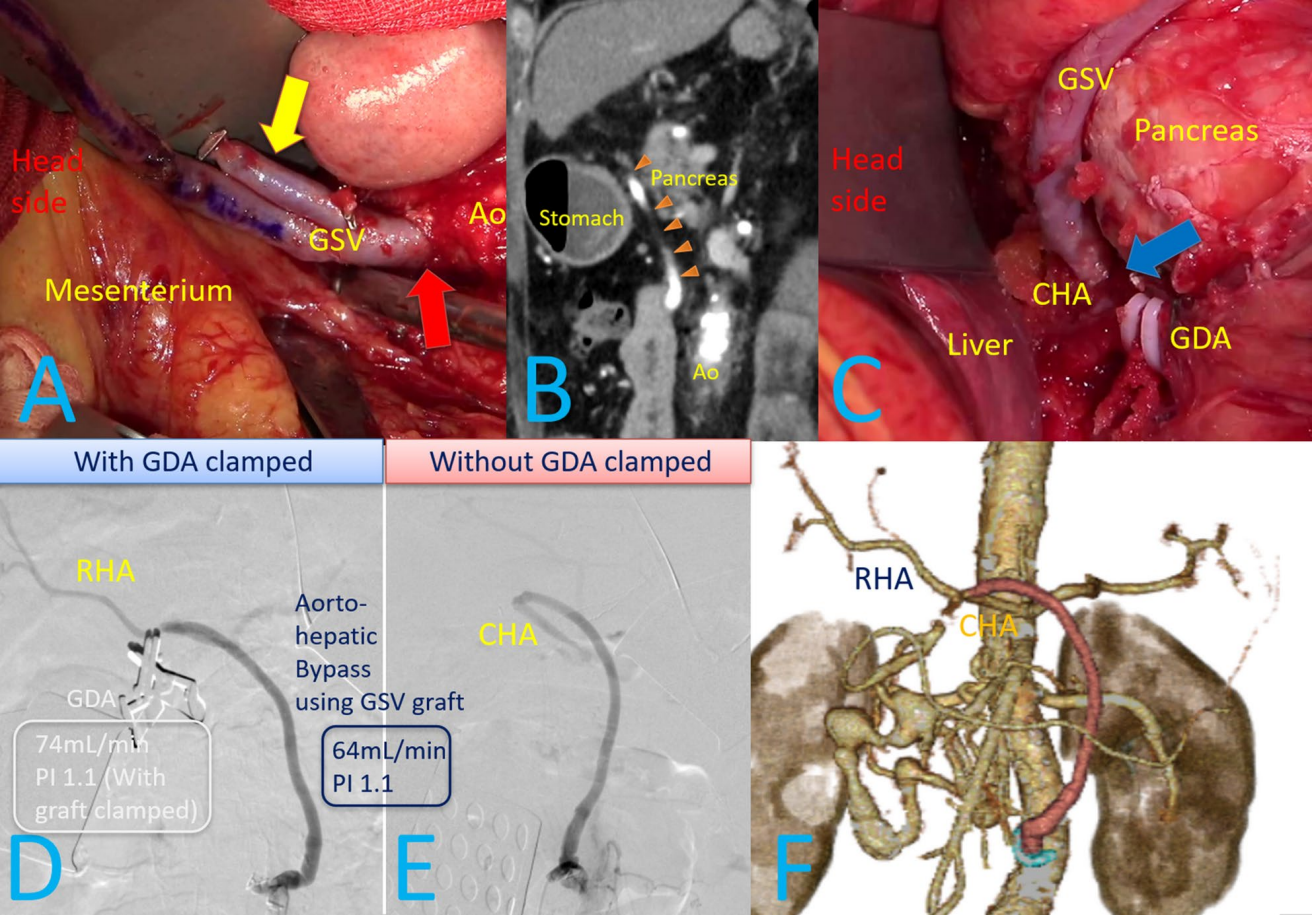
Intraoperative and postoperative findings. A A reversed great saphenous vein (GSV) graft (red arrow) anastomosed to the abdominal aorta, 15 mm above the inferior mesenteric artery, using an end-to-side parachute technique with 6-0 polypropylene. Yellow arrow is a branch of the GSV, which was preserved and used for intraoperative angiography. B To achieve the shortest route, the graft (orange arrowhead) was passed from the abdominal aorta to the common hepatic artery (CHA) through the mesocolon near the ligament of Treitz and between the stomach and pancreas. C The graft was anastomosed to the CHA (blue arrow) with an end-to-side parachute technique using 7-0 polypropylene. After angiography, the gastroduodenal artery (GDA) was ligated to prevent competitive flow between the GDA and the graft. D Angiography from the graft with the GDA clamped showed flow through the graft, right hepatic artery (RHA), and CHA. E Angiography from the graft without the GDA clamped showed flow only through the graft and CHA, with to-and-fro flow in the CHA. F Postoperative contrast-enhanced 3D CT demonstrated a patent aorto-hepatic bypass
